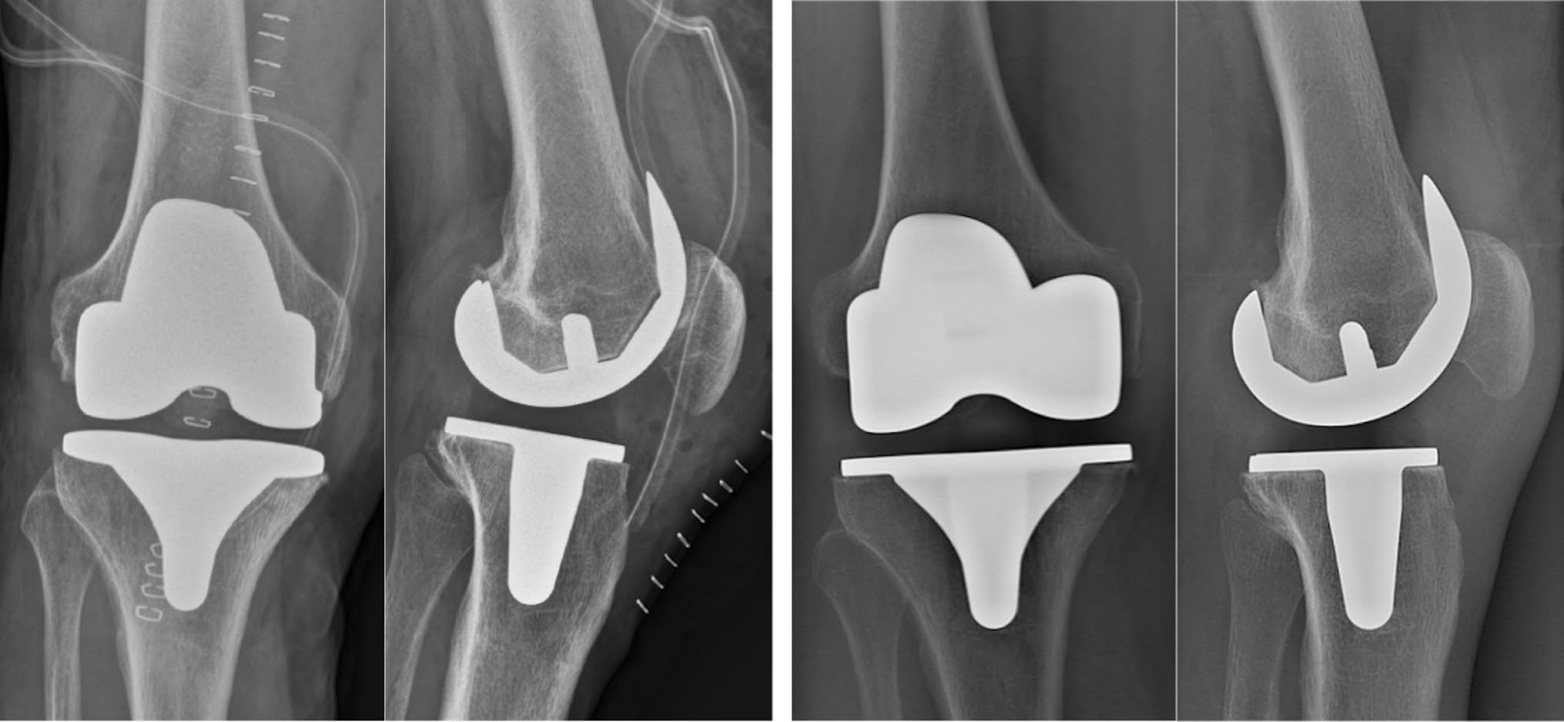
Temporary postoperative radiolucent lines in the lateral view. The X-rays on the left show the immediate postoperative result with a radiolucent line in Zone 3A at the lateral view with no residual radiolucent line in that zone at the X-ray 3 months after TKA in the same patient
